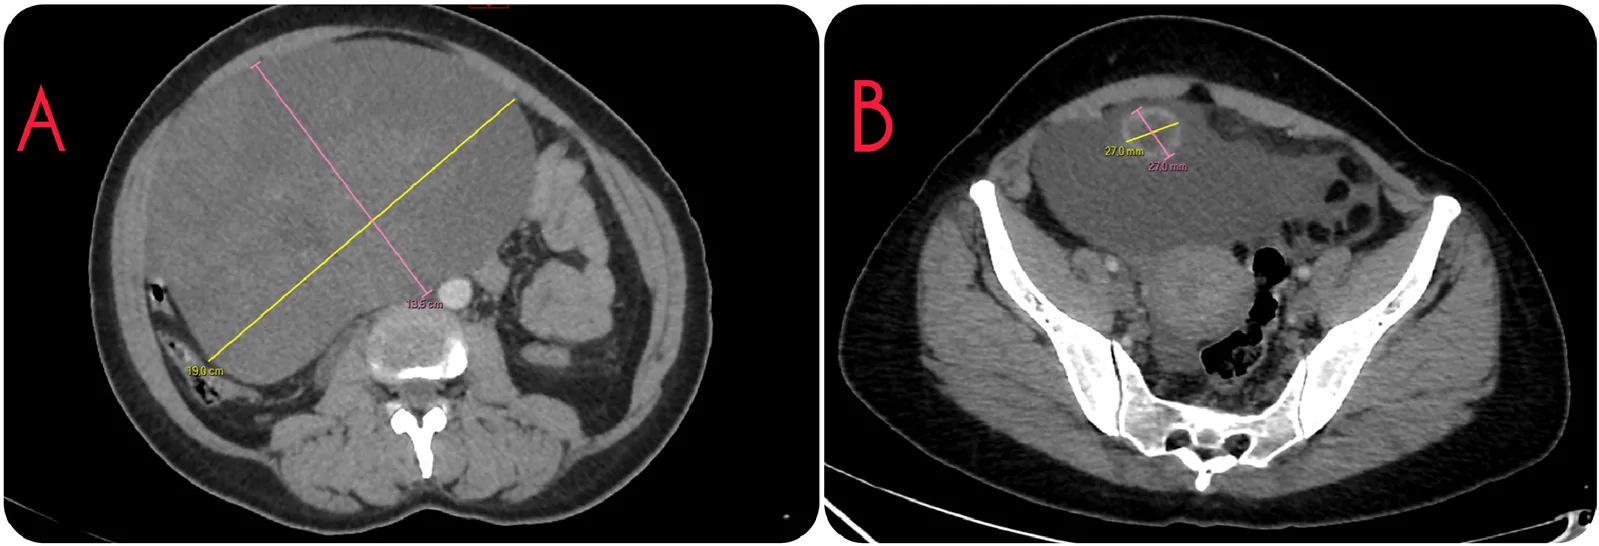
Axial contrast-enhanced CT images demonstrating the superior (A) and inferior (B) portions of the giant right ovarian mass. A nodular peritoneal lesion initially suspected for carcinomatosis is visible adjacent to the inferior aspect of the tumor.
